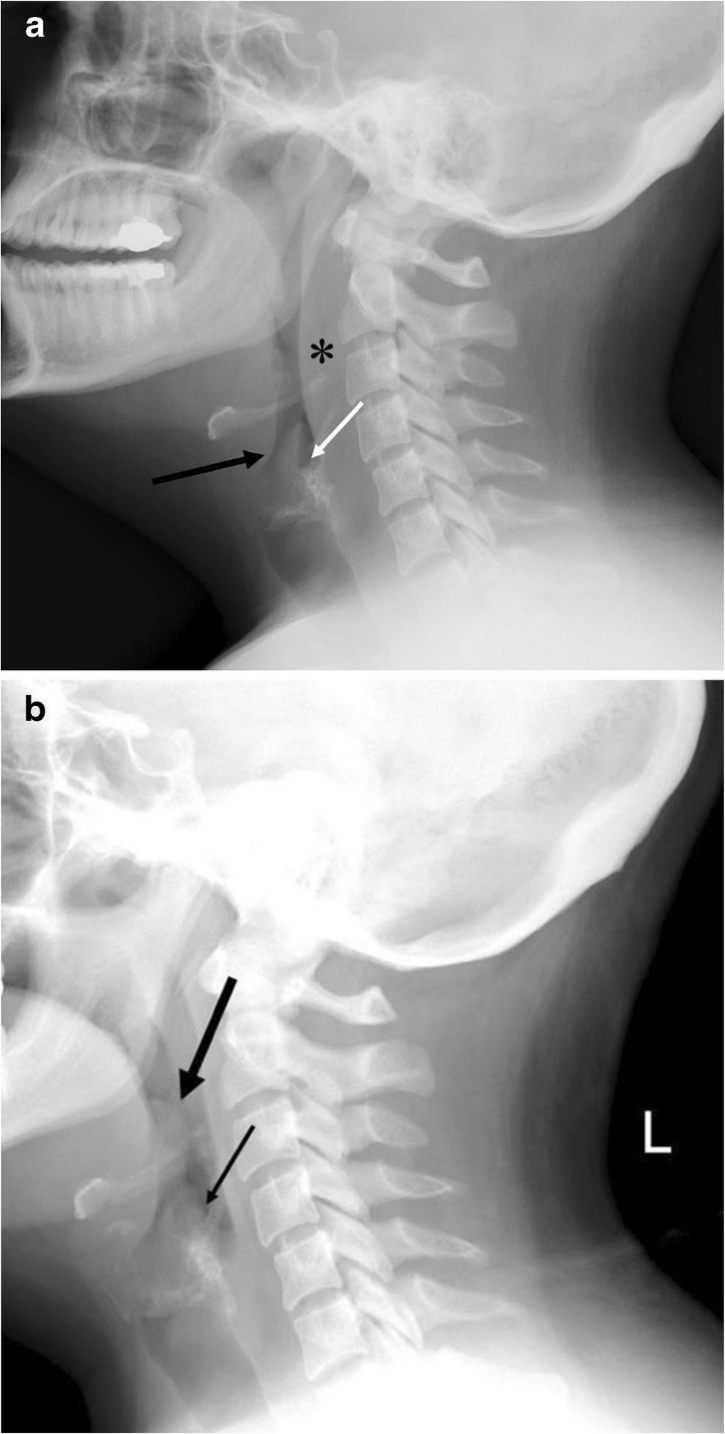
A 33-year-old female with hereditary angioedema (HAE). Soft tissue swelling of the neck during recurrent angioedema attacks. a The patient complained of neck swelling. Lateral radiograph of the neck shows thickening of the retropharyngeal soft tissue (*asterisk*), enlargement of the epiglottis with narrowing of the vallecula (*large arrow*), and thickening of the aryepiglottic folds (*small arrow*). b Six months later, the patient again complained of neck swelling and swallowing difficulty. Lateral view of the neck plain film shows enlargement of the epiglottis (*large arrow*, thumb sign) and thickening of the aryepiglottic fold (*small arrow*). No retropharyngeal soft tissue thickening is noted
